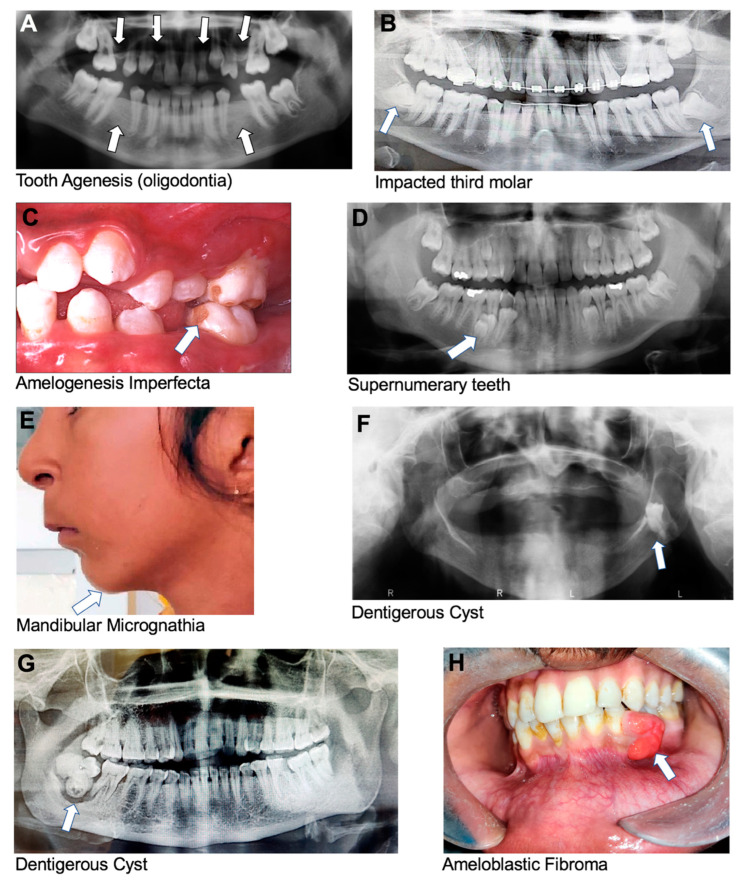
Oral conditions with strong genetic components as per our data analysis of retrieved articles. (A) Panoramic radiograph image of individual with oligodontia showing absence of six permanent teeth (excluding third molars). The following teeth are missing: 12, 15, 22, 25, 35, and 45, using the FDI numbering system (courtesy of Dr. Ariadne Letra). (B) Panoramic radiograph image of individual with impacted third molars. (C) Amelogenesis imperfecta showing enamel pitting with exposed dentin (courtesy of Dr. Cleverick (C.D.) Johnson). (D) Supernumerary teeth. (E) Mandibular micrognathia showing short lower jaw. (F,G) Dentigerous cyst (Image G is courtesy of Dr. Ritu Tiwari). (H) Ameloblastic fibroma at the lower left quadrant.
